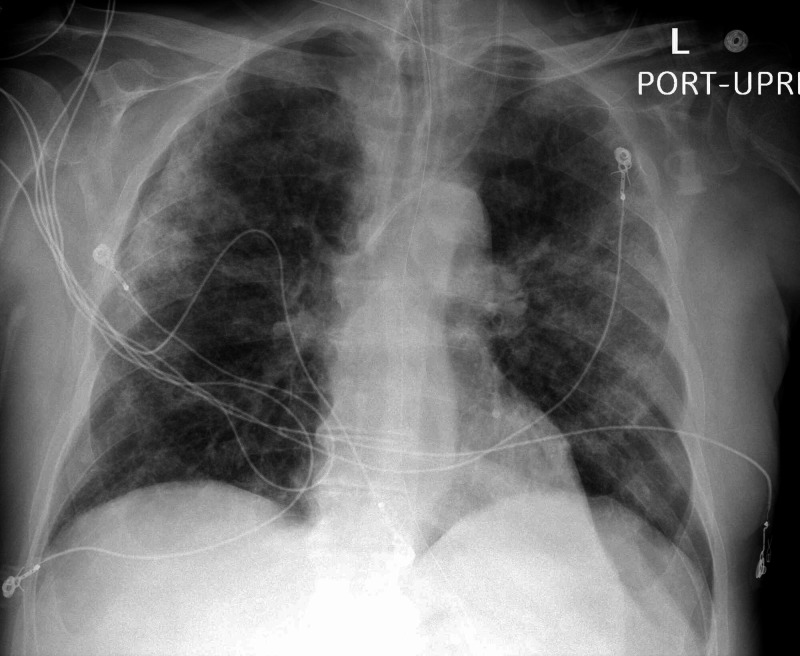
Chest x-ray of the patient indicating COVID-19 pneumonia
